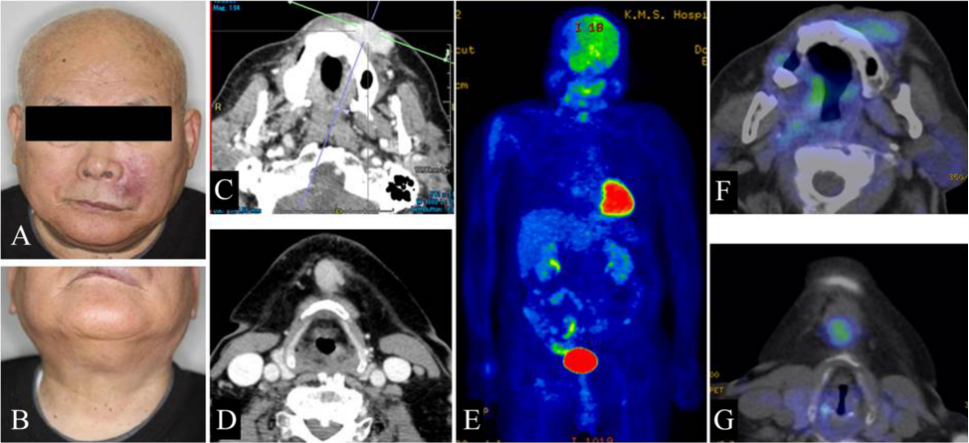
First medical examination. (A) Anterior face view. (B) Neck view. (C,D) Computed tomography of the head and neck region. (E,F,G) PET-CT of the whole body and head and neck region.
